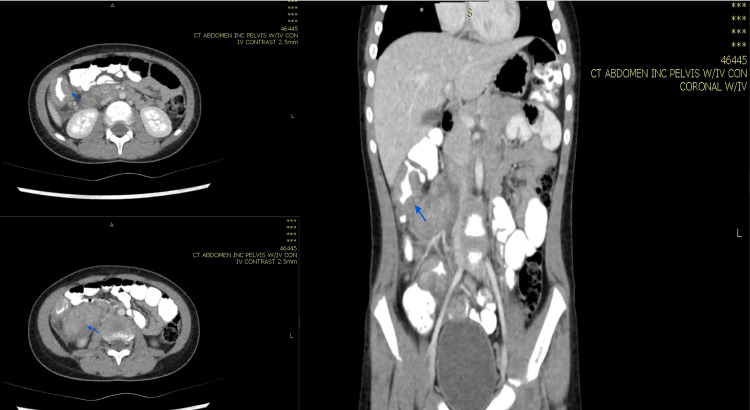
CT abdomen showing significant wall thickening of the ascending colon and terminal ileum with luminal narrowing (blue arrow), accompanied by multiple variable-sized lesions seen in the mesenteric fat of the right side of the abdomen suggestive of enlarged and pathological lymph nodes (the largest measuring approximately 40 × 23 mm).
